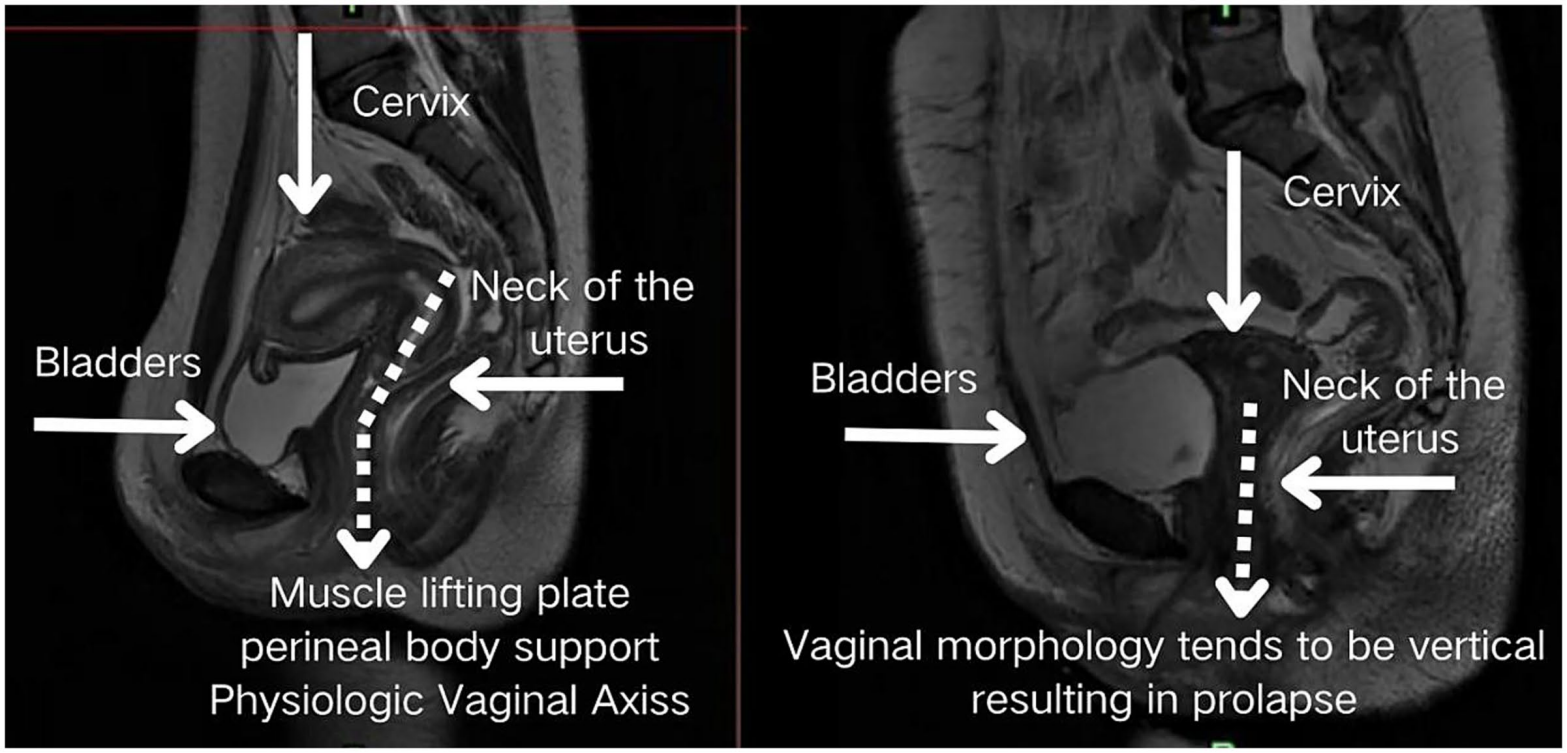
MRI validation of the piston-cylinder mechanism in a patient.
